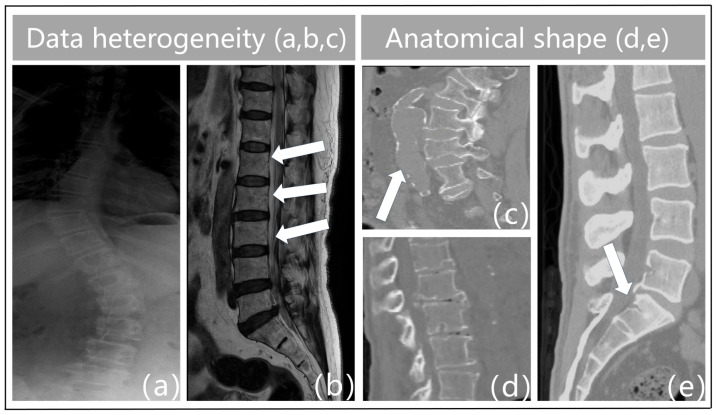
Challenges in resolving multi-modality vertebral images include: (a) boundary-blurring from X-ray imaging methods. (b) interclass similarity when vertebrae are present in MR. (c) The misdiagnosis in CT imaging of vertebrae and adjacent tissues with similar CT values. (d,e) weak vertebral contouring from the encroachment of vertebral degeneration/disease.
